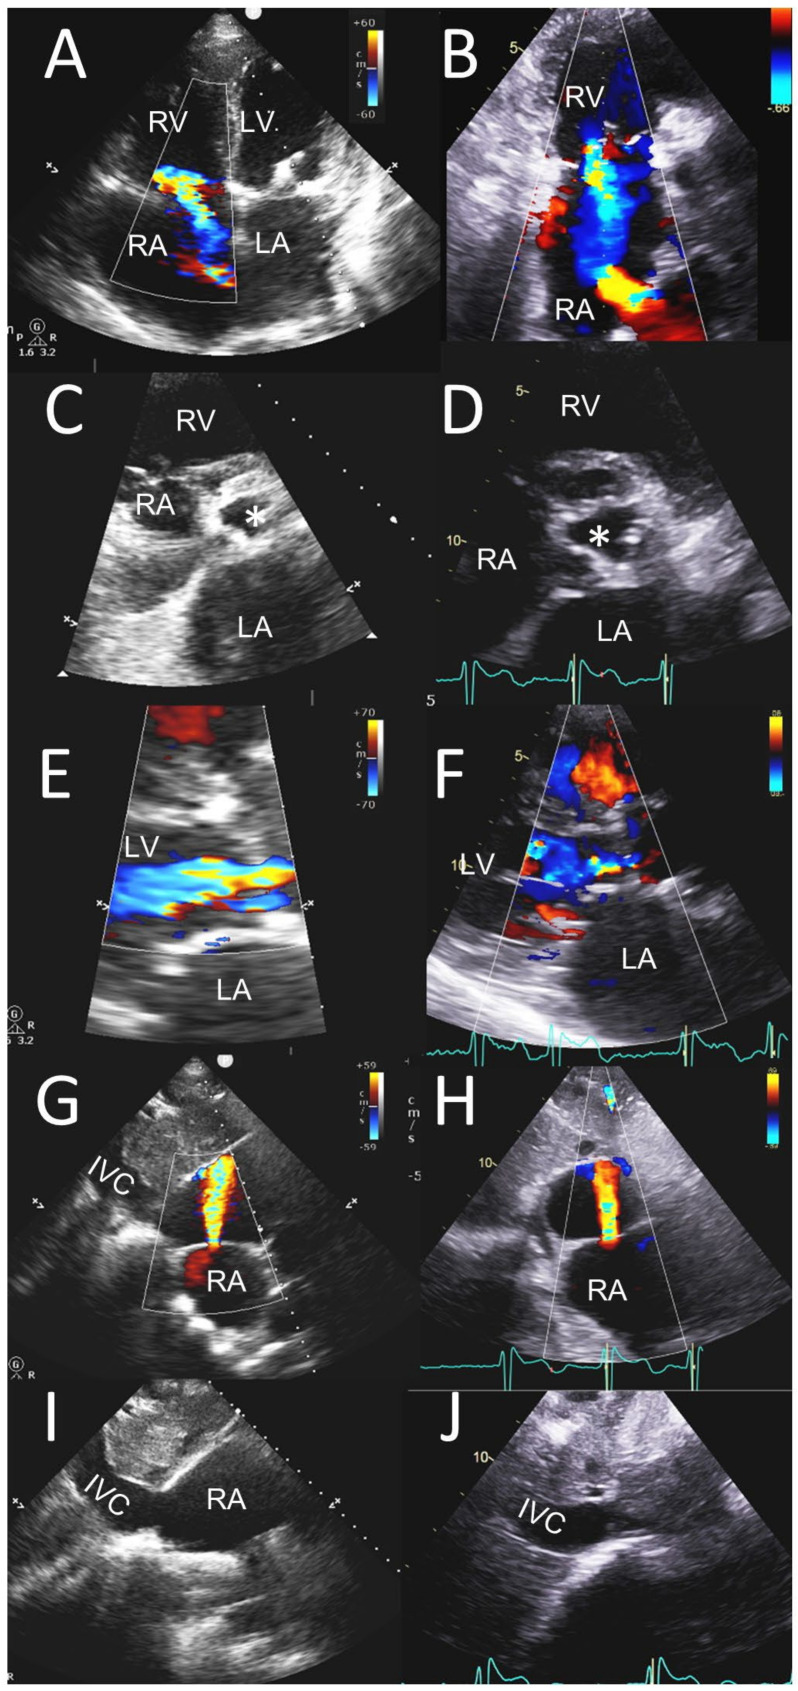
POCUS in assessment of chamber size, shunts and native VHD. Significant TR, AR and other findings. POCUS images (left-side panels) compared to the sTTE images (right-side panels) in the same patient as in Figure 1. (A,B) Tricuspid inflow views in four-chamber view (A) and basal short-axis view (B) showing moderately dilated RV and severely enlarged RA (A) with moderate appearing TR jet (A,B) and left-to-right flow across mid interatrial septum from prior trans-septal puncture during TEER (B). (C,D) Parasternal short-axis views showing a moderately sclerotic trileaflet aortic valve in systole (white asterisks) with enlarged LA. (E,F) Parasternal long-axis views showing AR jet appearing mild to moderate in panel (E) and mild in panel (F). (G,H) Subcostal views showing dilated LA and RA and interatrial septum with left-to-right interatrial shunt though the center of interatrial septum from prior TEER. (I,J) Subcostal sagittal views showing IVC. IVC, inferior vena cava; LA, left atrium; LV, left ventricle; RA, right atrium; RV, right ventricle.
